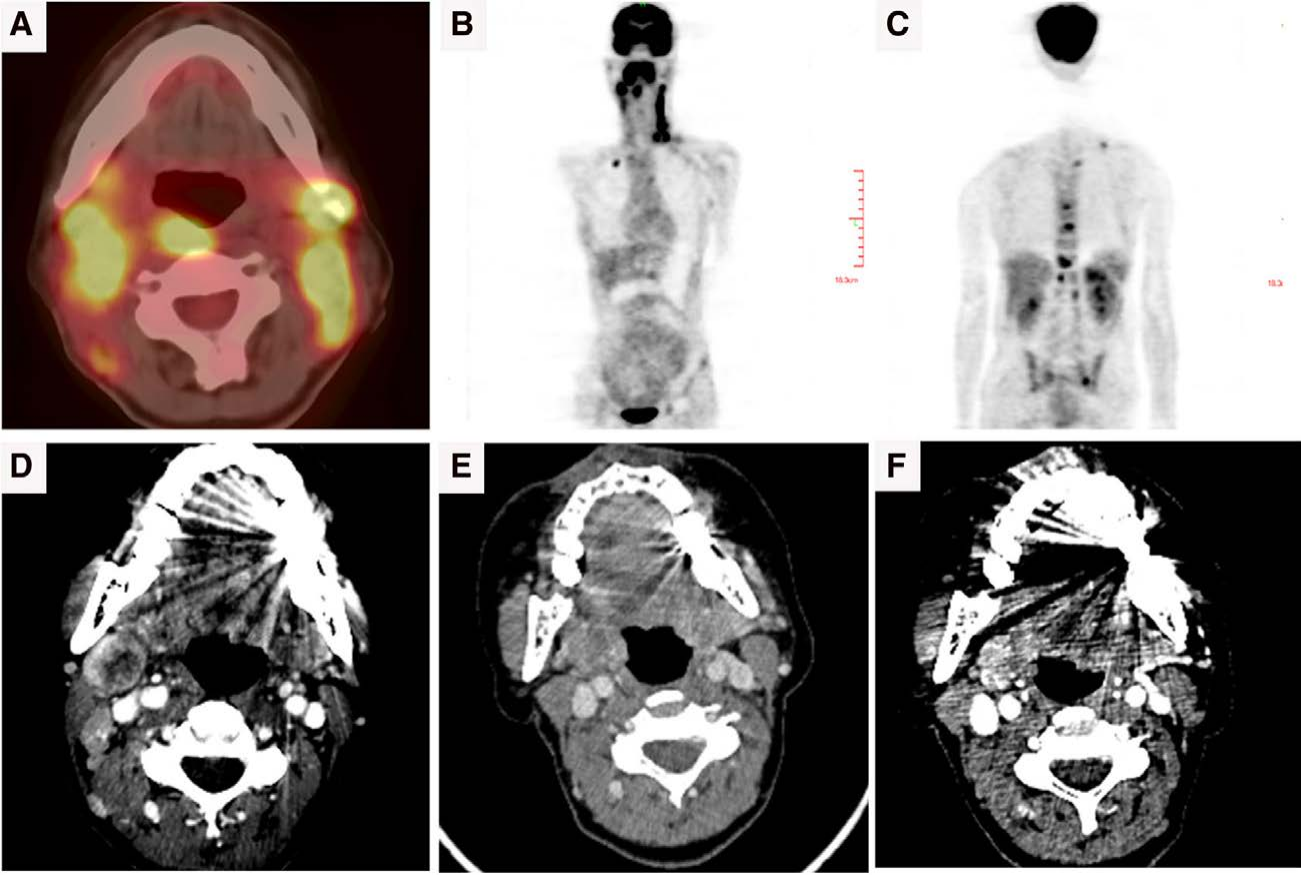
Images of case 2. (A)(B)(C) Significant 18F-FDG uptake in multiple sites on PET/CT scan, including soft tissues in the nasopharynx, right ethmoid sinus, soft palate, right oropharyngeal wall, and right tonsil, lymph nodes, bone and bone marrow, the maximum standardized glucose uptake value range from 6.9 to 8.3. Changes of the lymph node in the right neck on enhanced CT scan, including enlarged lymph node with necrosis in the first visit (about 2.5 cm × 2.2 cm) (D), stabled lymph node after P-Gemox chemotherapy (about 1.8 cm × 1.9 cm) (E), reduced lymph node (about 1.6 cm × 1.7 cm) after 5 cycles of immunotherapy (F). FDG = fluorodeoxyglucose, PET/CT = positron emission tomography-computed tomography.
